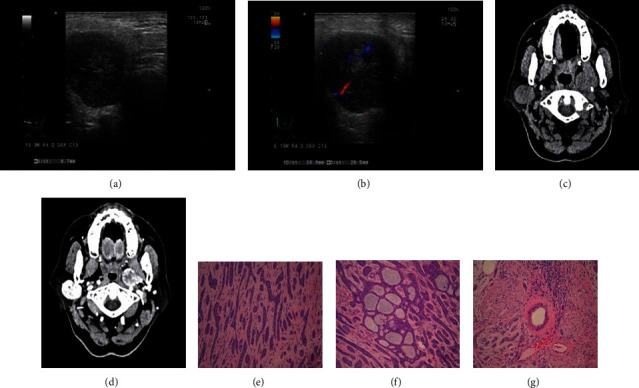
A 68-year-old woman with a trabecular-type BCA. (a) A 68-year-old woman with a regular, ill-defined, hypoechoic, heterogeneous mass without posterior enhancement in the right parotid gland. (b) Intramodular prominent vascularity on Doppler B. (c) Plain computed tomography scan showing a regular, ill-defined mass with uneven low density. One of the trabecular type with extensive cribriform structures. (d) Computed tomography angiography (CTA): continuous heterogeneous enhancement in the arterial and venous phases. (e) (trabecular) neoplastic basal-like cells are arranged in a cord-like structure (×200). (f) Trabecular mass with extensive cribriform structures (×200); Scar bar. (g) Tumor cells invading the nerves (×100).
